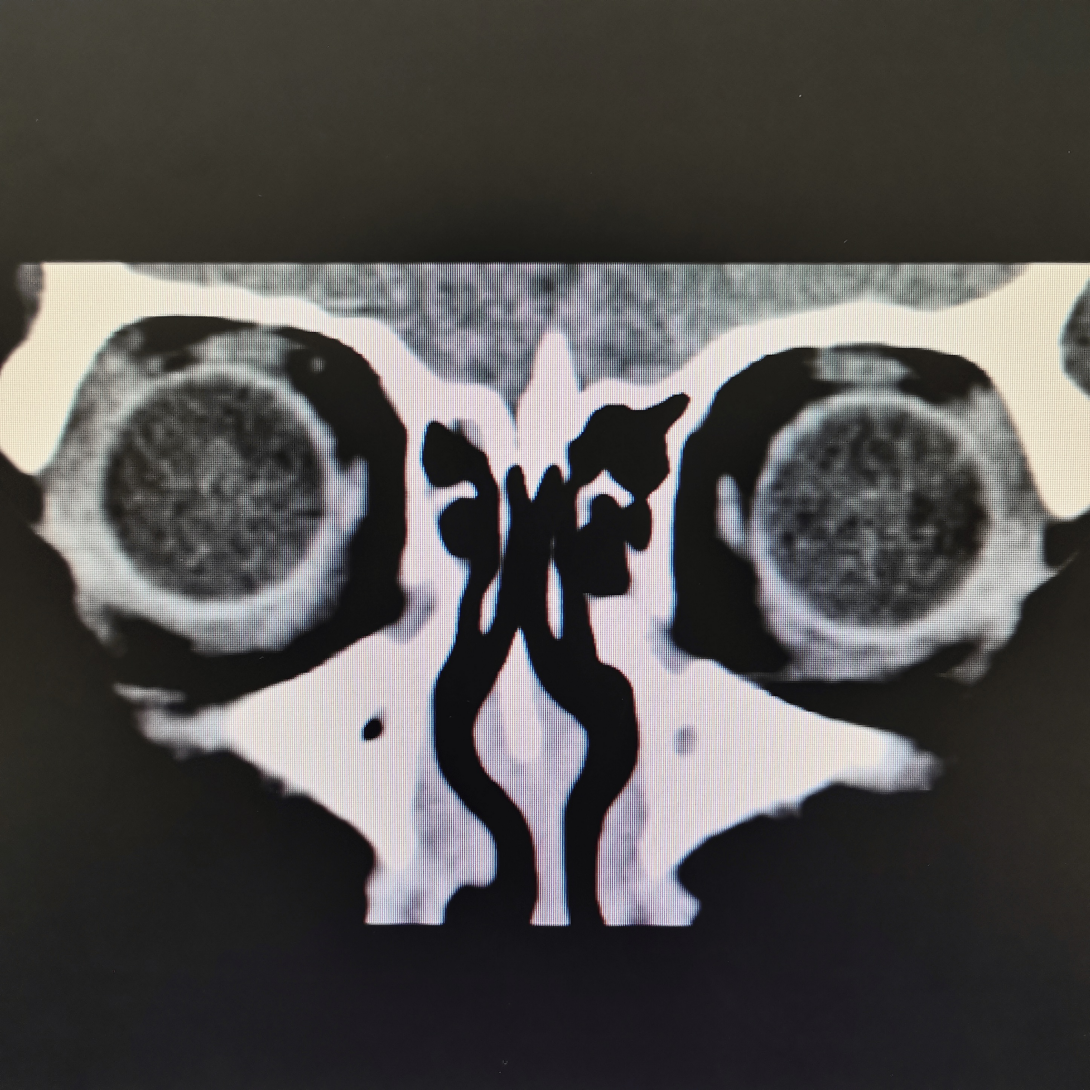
CT brain + ocular (coronal view) shows no significant abnormalities
